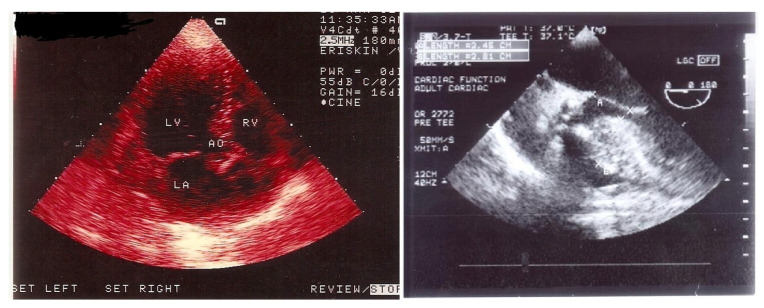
Thickened septal base on the transthoracic echocardiography in a patient with AS (LV: Left ventricle, RV: Right ventricle, LA: Left atrium, AO: Aortic valve outflow tract) (left) and the severely thickened septal base by the transesophageal echocardiography in another patient with severely stenotic AS (right).
